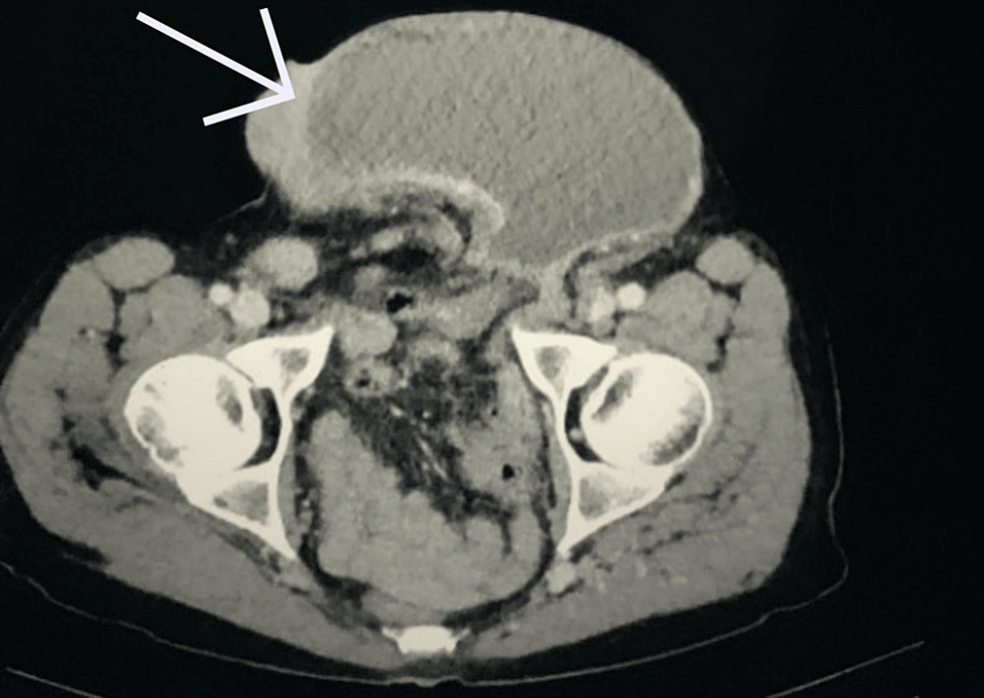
Computed tomography of abdomen and pelvis showing dilated stomach within left inguinal hernia sac. Arrow pointing toward stomach within left inguinal canal.
